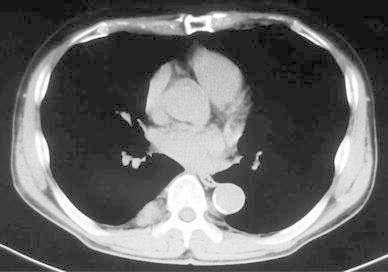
Chest CT shows a tumor located in the right posterior mediastinum. It is 2.5 cm in diameter beside the T8 and T9 thoracic vertebrae
